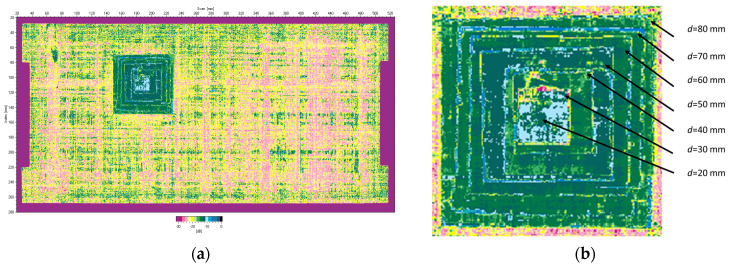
Ultrasonic C-scan (time-of-flight mode) of the plate with artificial damage scanned from the bottom side: (a) full scan; (b) zoom-in on the delamination.
